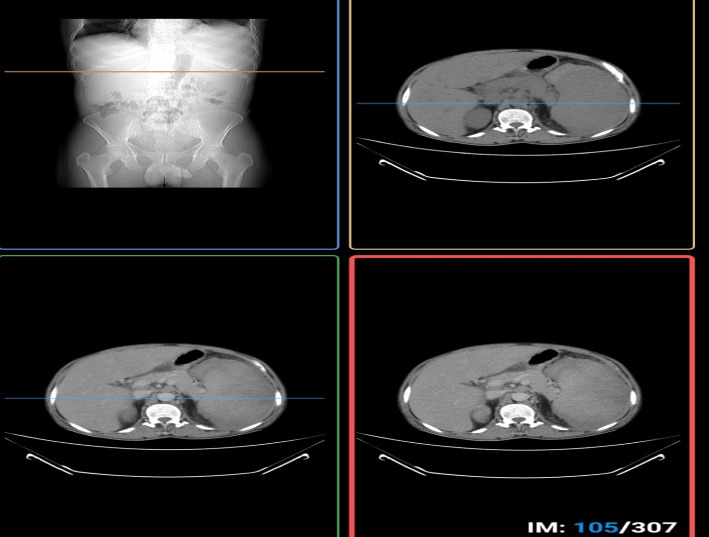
Abdominopelvic CT showing hepatomegaly and splenomegaly with subcapsular hematoma.
